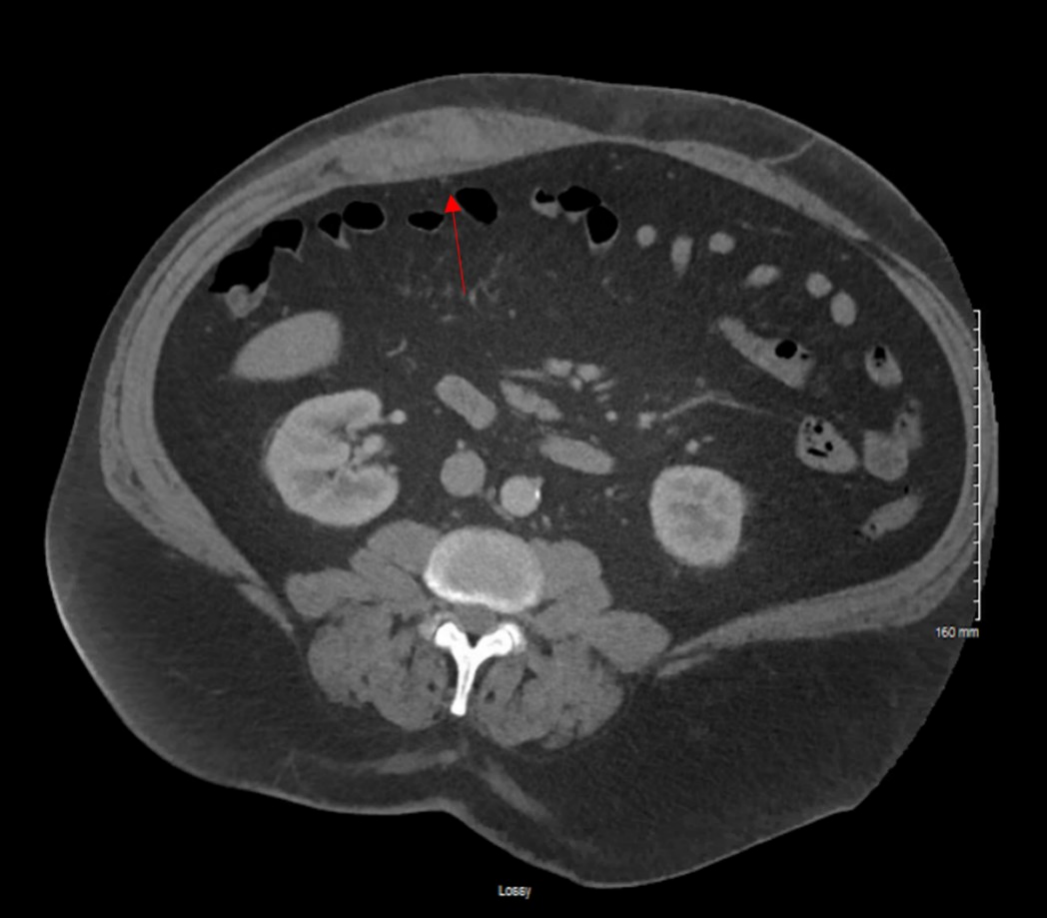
Figure 3. Transverse cut of CT imaging revealing right abdominal wall hematoma (red arrow) that was measured to be approximately 11.4 x 2.6 x 8.4cm in volume.
